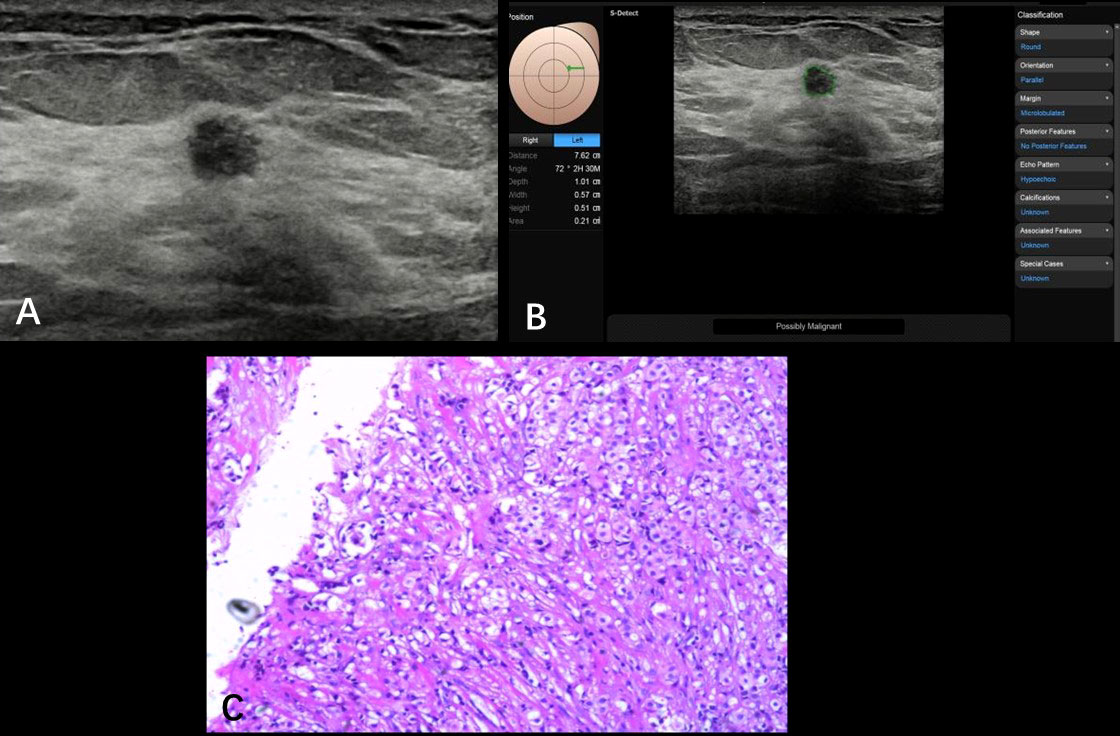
Non-specific invasive carcinoma from a 69-year-old patient (BI-RADS 4a). A hypoechoic nodule (0.57 × 0.51 cm) was found at the 2 o’clock point of her left breast; the ultrasound graphic showed an uneven edge, irregular shape, and uniform echo (A). S-Detect artificial intelligence recommendation was “possibly malignant” and the BI-RADS score receded from 4a to 4b (B). Pathologically confirmed as breast non-specific invasive carcinoma (C).
